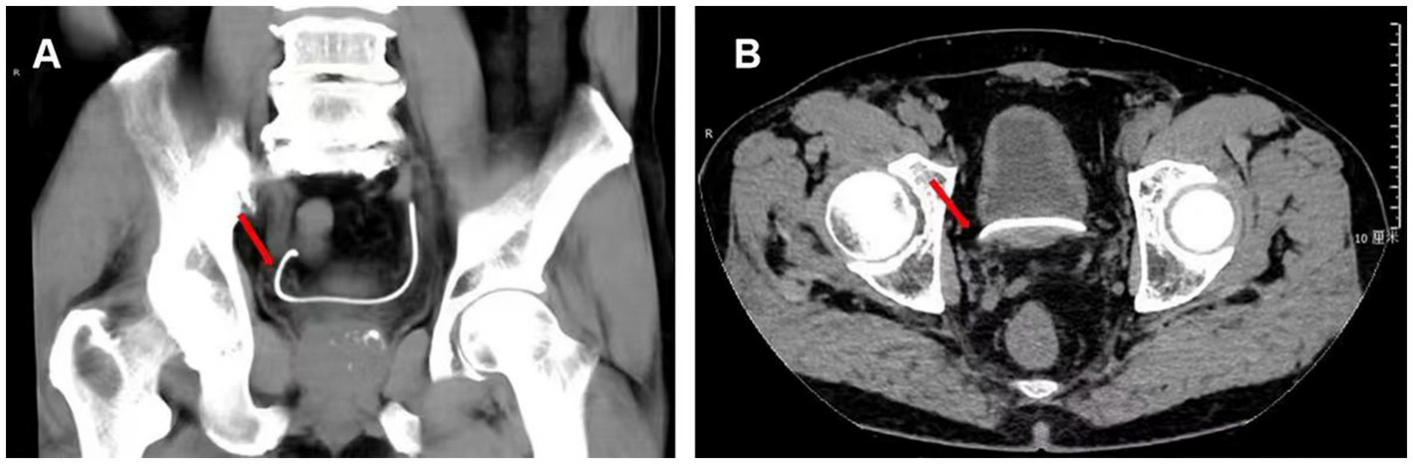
CT examination after PCNL revealed that the distal end (red arrow) of the left ureteral stent was displaced beyond the bladder and into the right ureter. (A) Coronary section. (B) Axial section.
